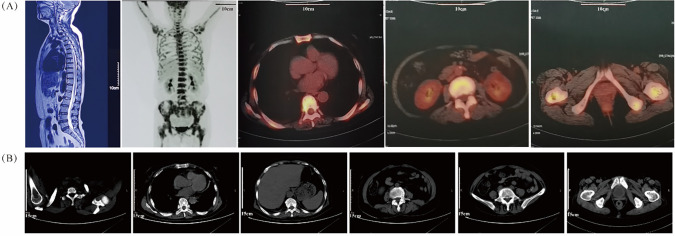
Radiologic results by MRI, PET/CT and CT scans. (A) The MRI image (left panel) shows multiple abnormal bone signals in the vertebral column. The PET/CT images (right four panels) reveal extensive uptake of bone marrow without evidence of involvement in the liver, spleen, or lymph nodes; (B) The plain CT scans before treatment do not show any evident bone lesions
